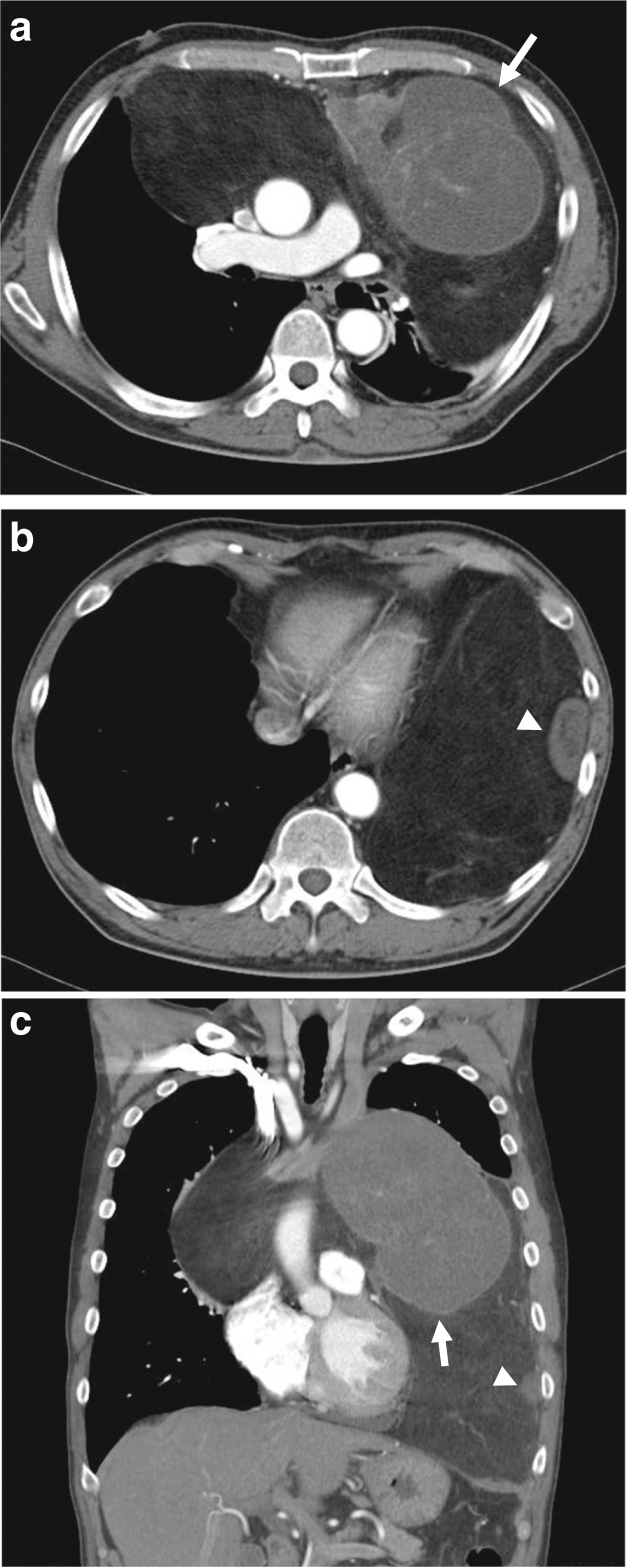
Chest CT images showing a a well-circumscribed mass in the anterior mediastinum measuring 36 cm × 18 cm × 10 cm and compressing the lower lobes bilaterally, b the tumor is composed mostly of fatty tissue but contains two solid areas, and c the left brachiocephalic vein is circumferentially surrounded by tumor. In these figures, the *arrow* and *arrowhead* showed each solid area
